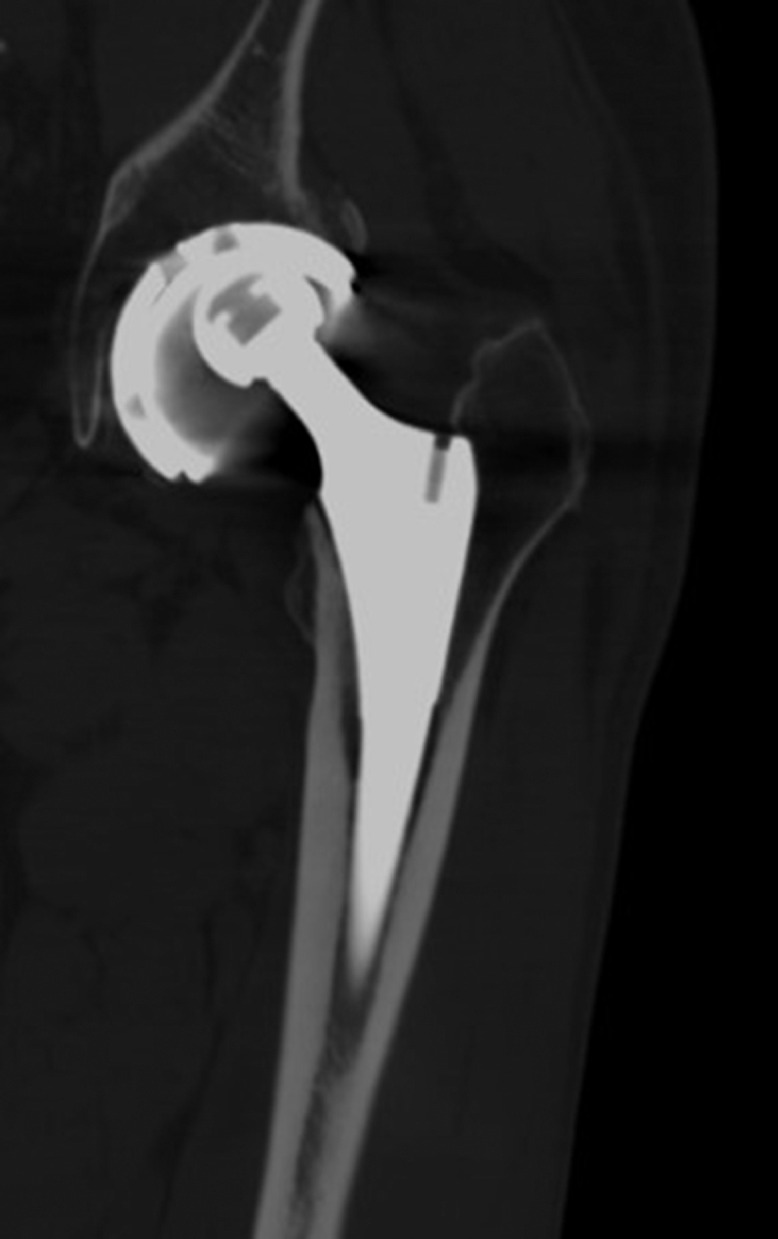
Coronal CT image of the left hip confirms eccentric positioning of the ceramic femoral head within the superior aspect of the metal shell and acetabular cup assembly. In this CT image, the ceramic femoral head articulates with the metal shell without an intervening polyethylene femoral head component. The metal shell is within the acetabular cup.
